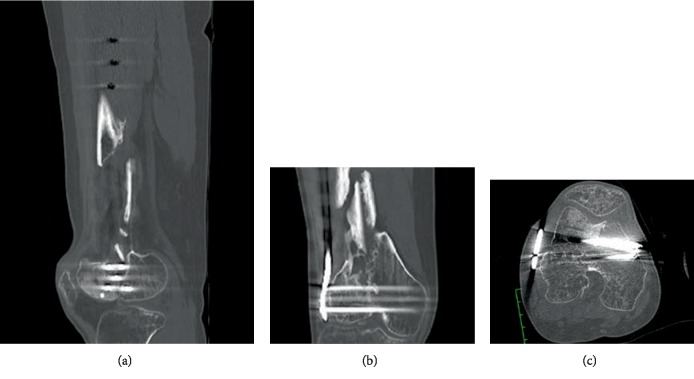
Preoperative CT scan of the right knee. Sagittal (a), coronal (b), and axial (c) CT scan shows the step-off between articular fragments.
